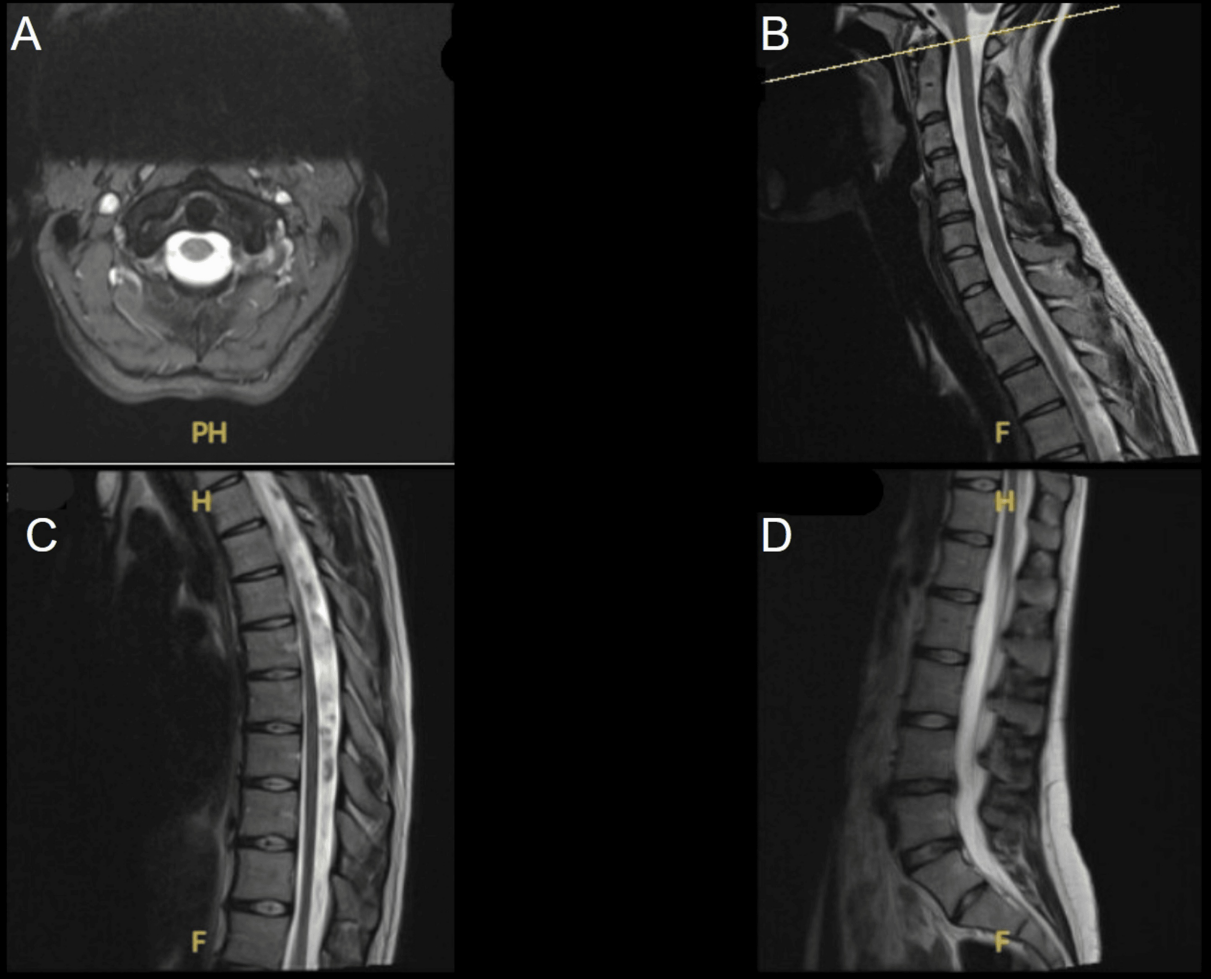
MRI of the spine (A–D) showing the cervical, thoracic and lumbar regions. No evidence of focal lesion. No findings to suggest inflammatory/demyelinating pathology.
